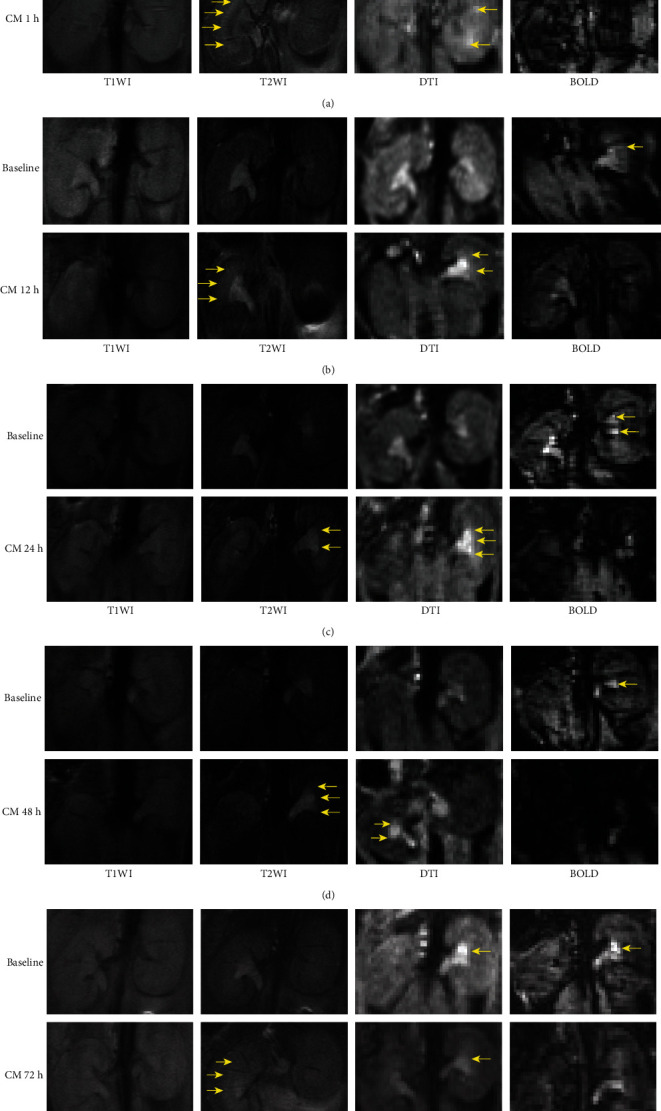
Representative kidney MRI imaging (a–e). The arrows show the alterations related to injection of CM. No obvious alterations present on T1WI. Significant enhancing signal alterations present on T2WI and DTI. Some signal-associated oxygen contents disappear on BOLD imaging after CM administration. CM: contrast media; DTI: diffusion tensor imaging; BOLD: blood oxygen level-dependent imaging; T1WI: T1-weighted image; T2WI: T2-weighted image.
